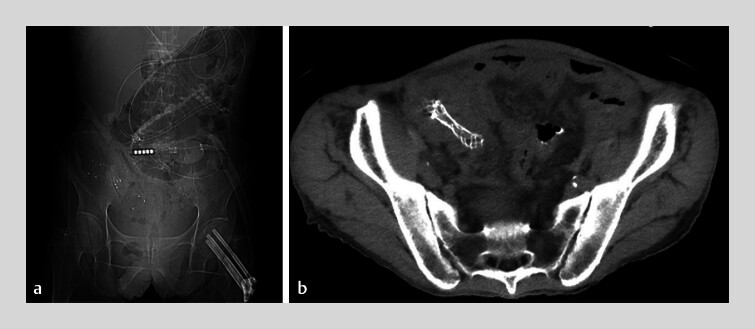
Radiographs taken 1 day post-stent placement.
